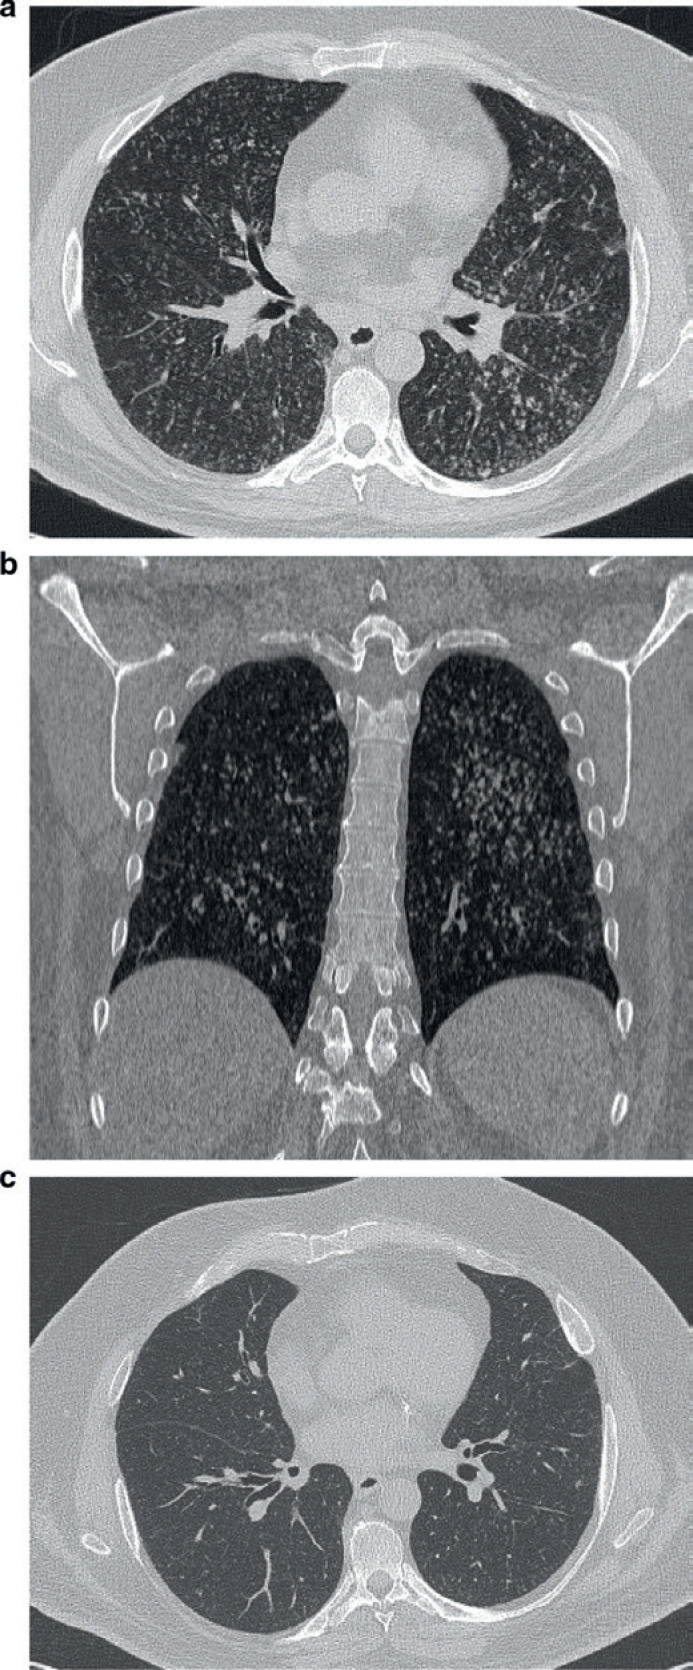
HRCT. (a) Das axiale Thorax-CT zeigt multiple kleine, unscharfe noduläre Verdichtungen mit diffuser Verteilung über beide Lungenfelder. (b) Das Thorax-CT in der Frontalebene zeigt ein *Tree-in-Bud*-Muster im linken unteren Lungenfeld. (c) Das axiale Thorax-CT drei Monate nach der stationären Aufnahme zeigt eine Reduktion der multiplen nodulären Verdichtungen.
